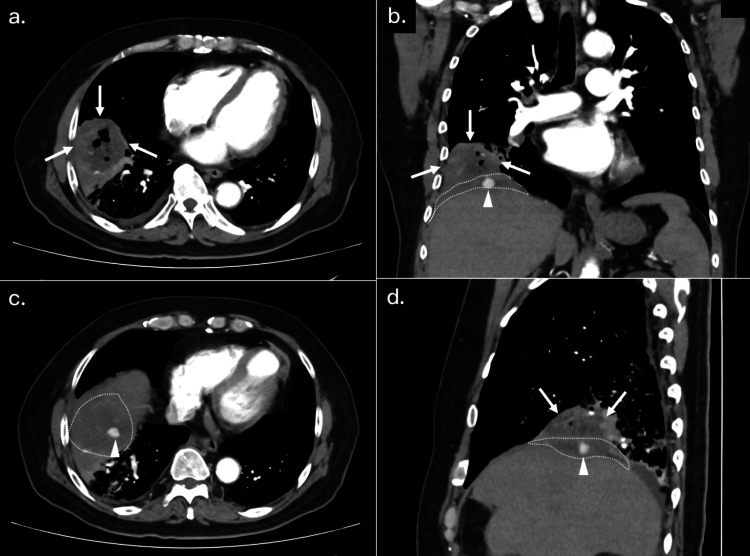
CT scan findings when hemoptysis appeared. (a-d) A contrast-enhanced CT, when the patient presented with fever, wet cough, and hemoptysis, revealed a 70 x 75 mm lung abscess (arrows) and pneumonia in the right lower lobe. The residual gallstone (arrowheads) was in the subphrenic abscess cavity (dotted areas).
